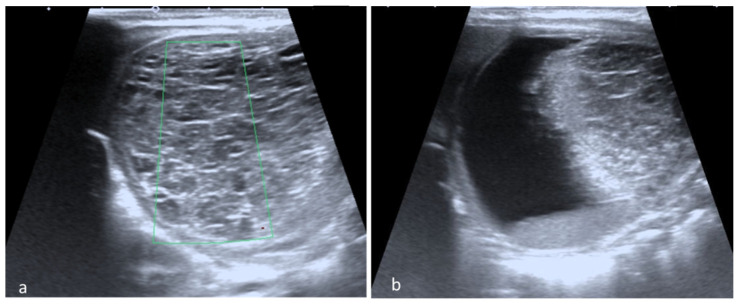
Ovarian torsion. (a) Postnatal abdominal ultrasound with enlarged, inhomogeneous structure without vascular Doppler signal; and (b) a cyst with fluid-fluid level.
